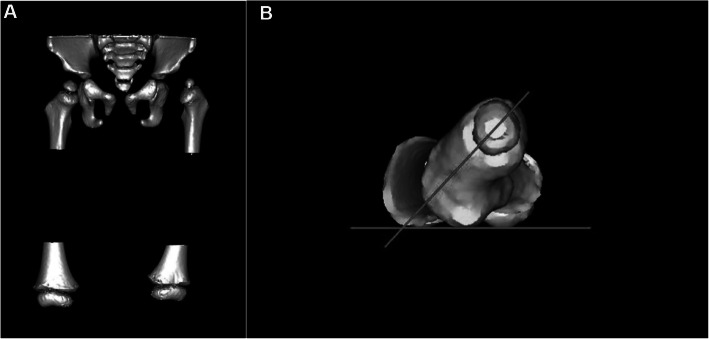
3D-FNA. a CT-reconstruction image of the pelvis and the distal and proximal femur. b 3D-FNA: the lowest point of the greater trochanter and the medial and lateral femoral condyles are located on a horizontal line by rotating the image. The angle between this horizontal line and the femoral neck axis is the 3D-FNA
